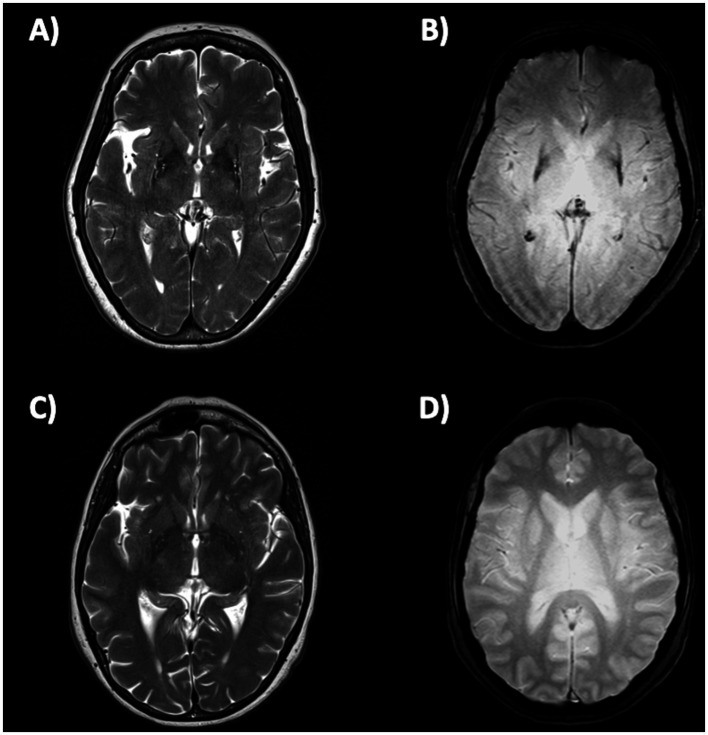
Brain axial 3T-MR images showing atrophy (A) and hypointensity (B) of postero-lateral putamina in multiple system atrophy—parkinsonian type patient, and the normal putaminal appearance in a patient with Parkinson’s disease (C,D). Figures (A–C) are T2-weighted axial MR images; figures (B–D) are T2*-weighted gradient echo (T2* GRE) axial MR images.
